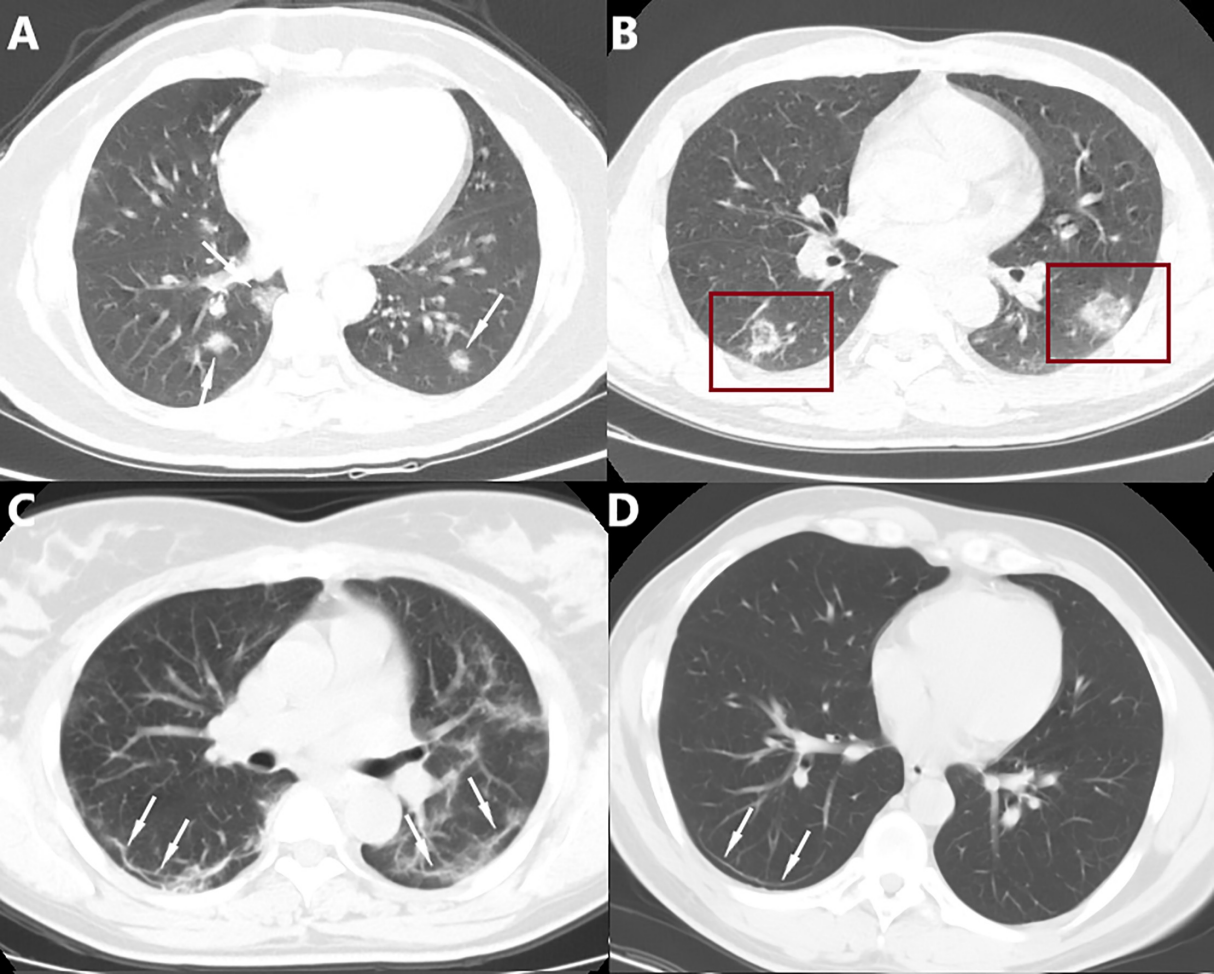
Relatively atypical CT manifestations. A. Multifocal solid irregular nodules (white arrow) with well- or poorly defined edges are shown in the subpleural area of the inferior lobes. B. A reversed halo sign (red frame) was in the subpleural area of the inferior lobes. C. Subpleural curvilinear opacities with poorly defined edges paralleling the pleural surface are shown in the inferior lobes. D. A subpleural thin curvilinear opacity with well-defined edges paralleling the pleural surface in the inferior lobe.
